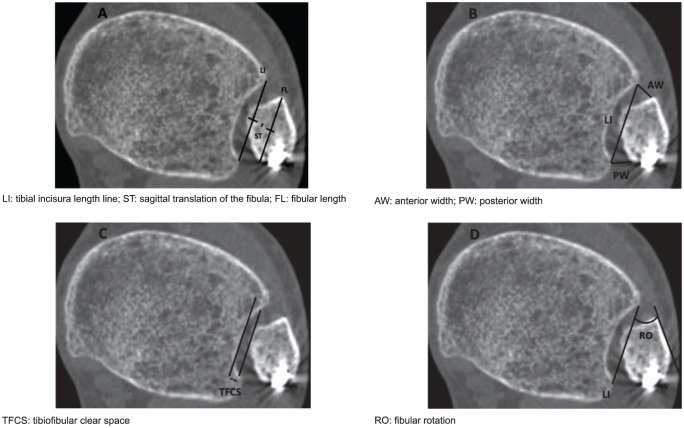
(A-D) Radiographic measurements.
